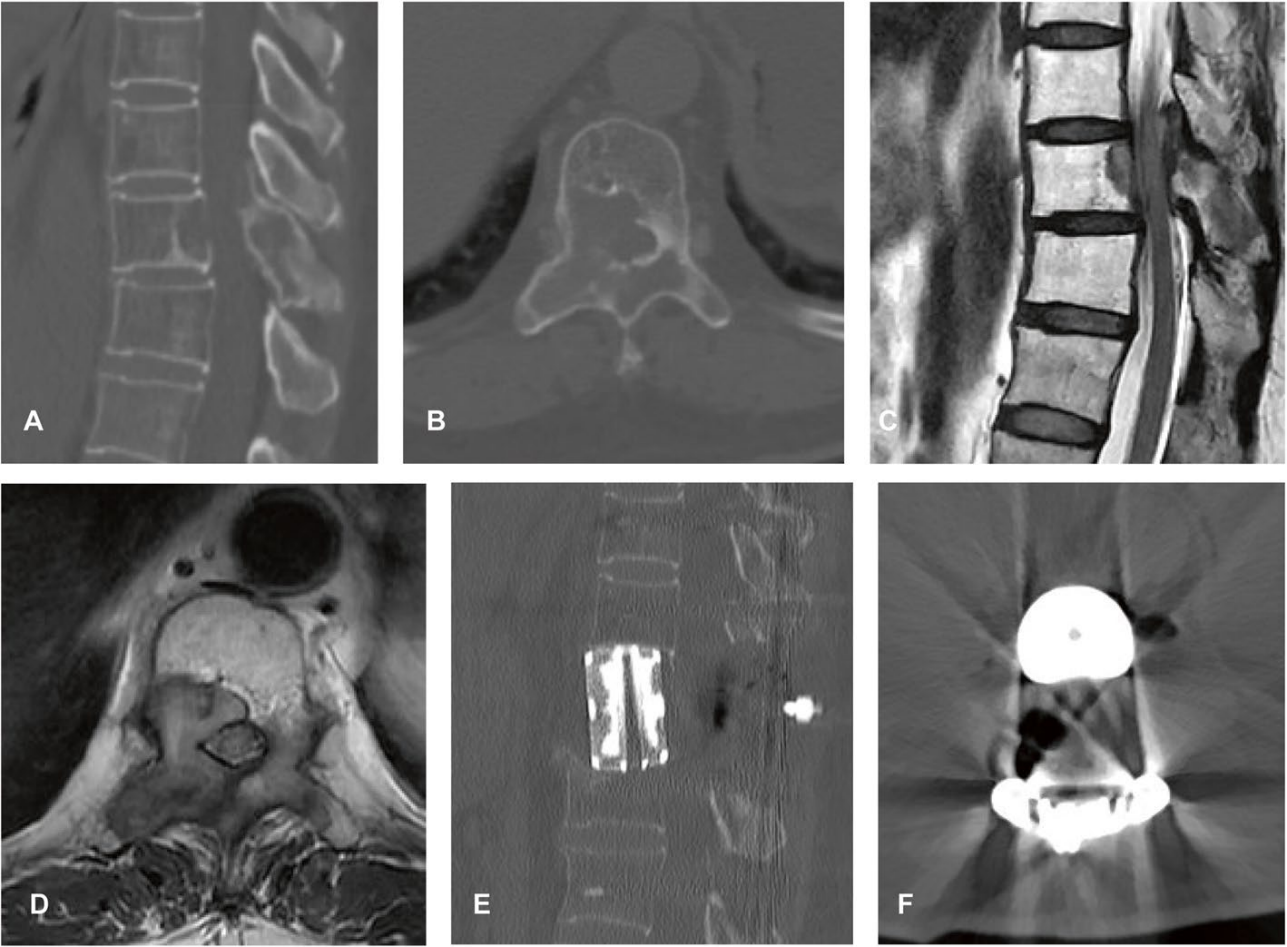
A 55-year-old woman with metastatic breast cancer experienced progressive weakness in the lower extremities for 3 months, her KPS score was 90, and the American Spinal Injury Association impairment scale was E. The patient underwent TES and spine reconstruction with off-the-shelf 3D-printed AVB via a posterior approach and the estimated blood loss was 1000 mL. The patient recovered well and no surgical complications occurred. A, B Preoperative CT scan showing the osteolytic body destruction of T10. C, D Sagittal and axial T2-weighted MRI showing epidural spinal cord compression. E, F Postoperative CT scan. Abbreviations: KPS, Karnofsky performance status; TES, total en bloc spondylectomy; 3D, three-dimensional; AVB, artificial vertebral body; CT, computed tomography; MRI, magnetic resonance imaging
